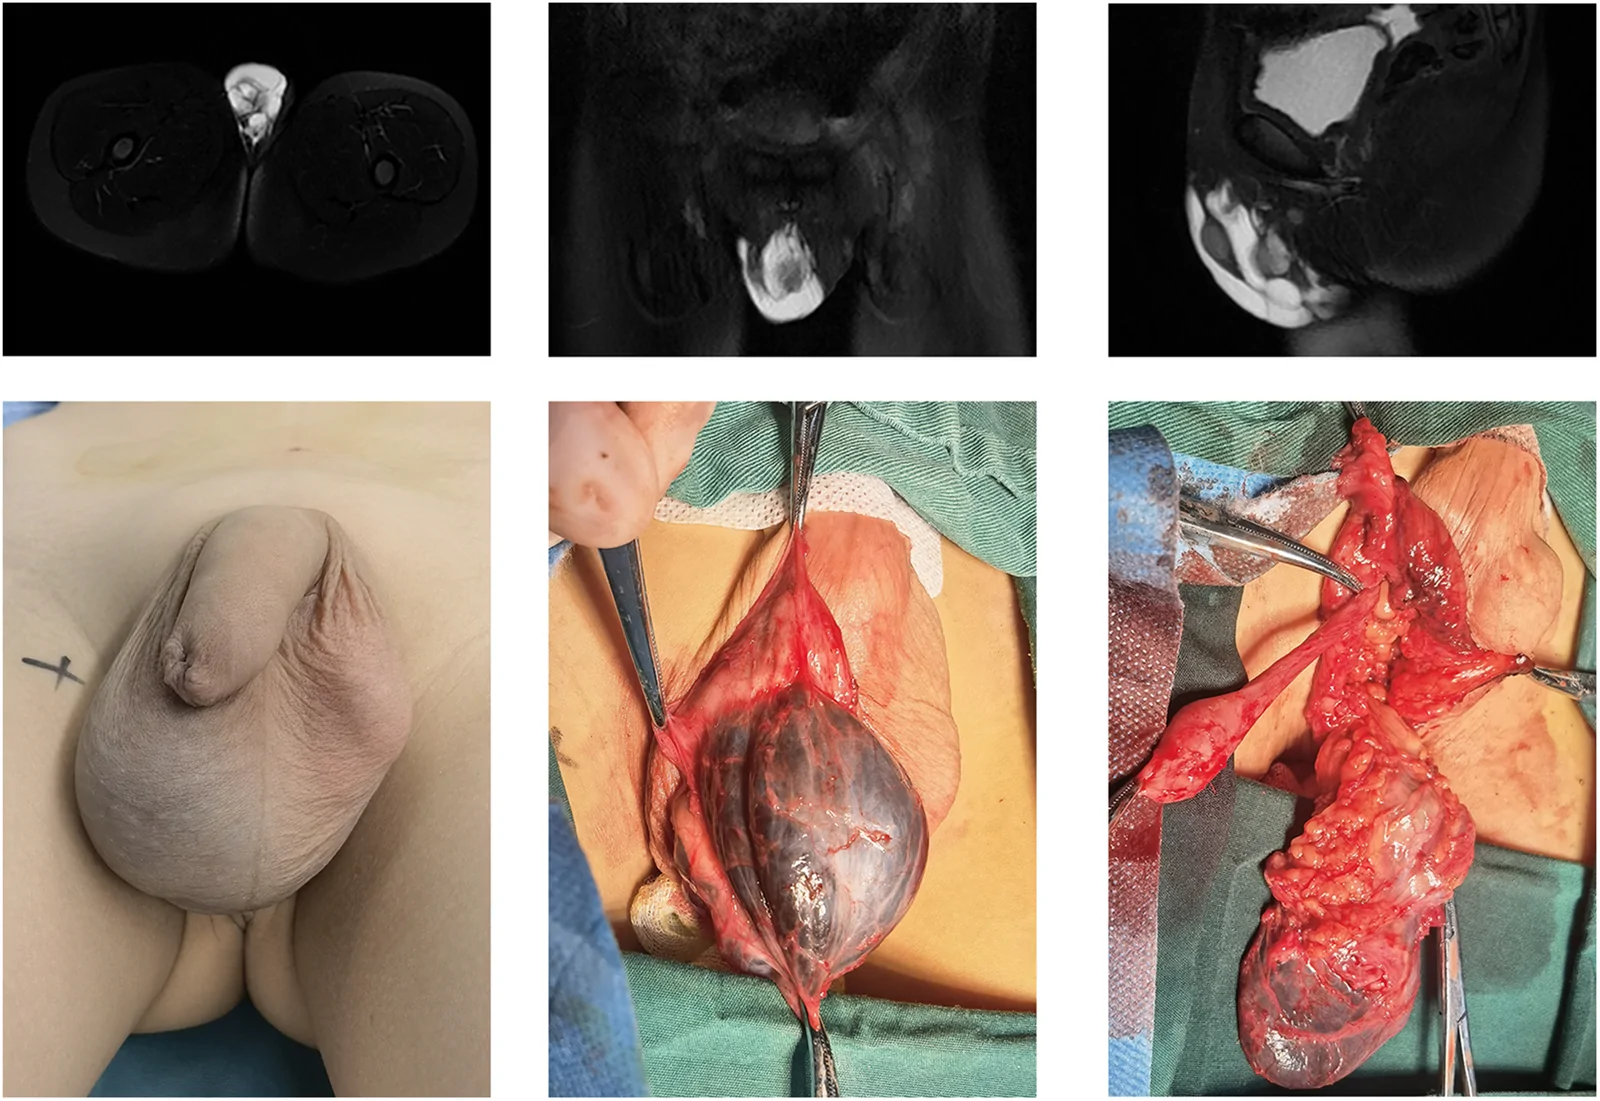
A representative example (case 5): (A–C) axial, coronal, and sagittal T2-weighted MRI images demonstrate a multiloculated cystic lesion of the scrotum with internal septations; (D) preoperative photograph of the scrotum; (E) the lesion completely encapsulates the tunica vaginalis and testis; (F) the tunica vaginalis is carefully dissected out, revealing diffuse lesion infiltration towards the scrotal septum, bulbar urethra, and pubic tubercle.
